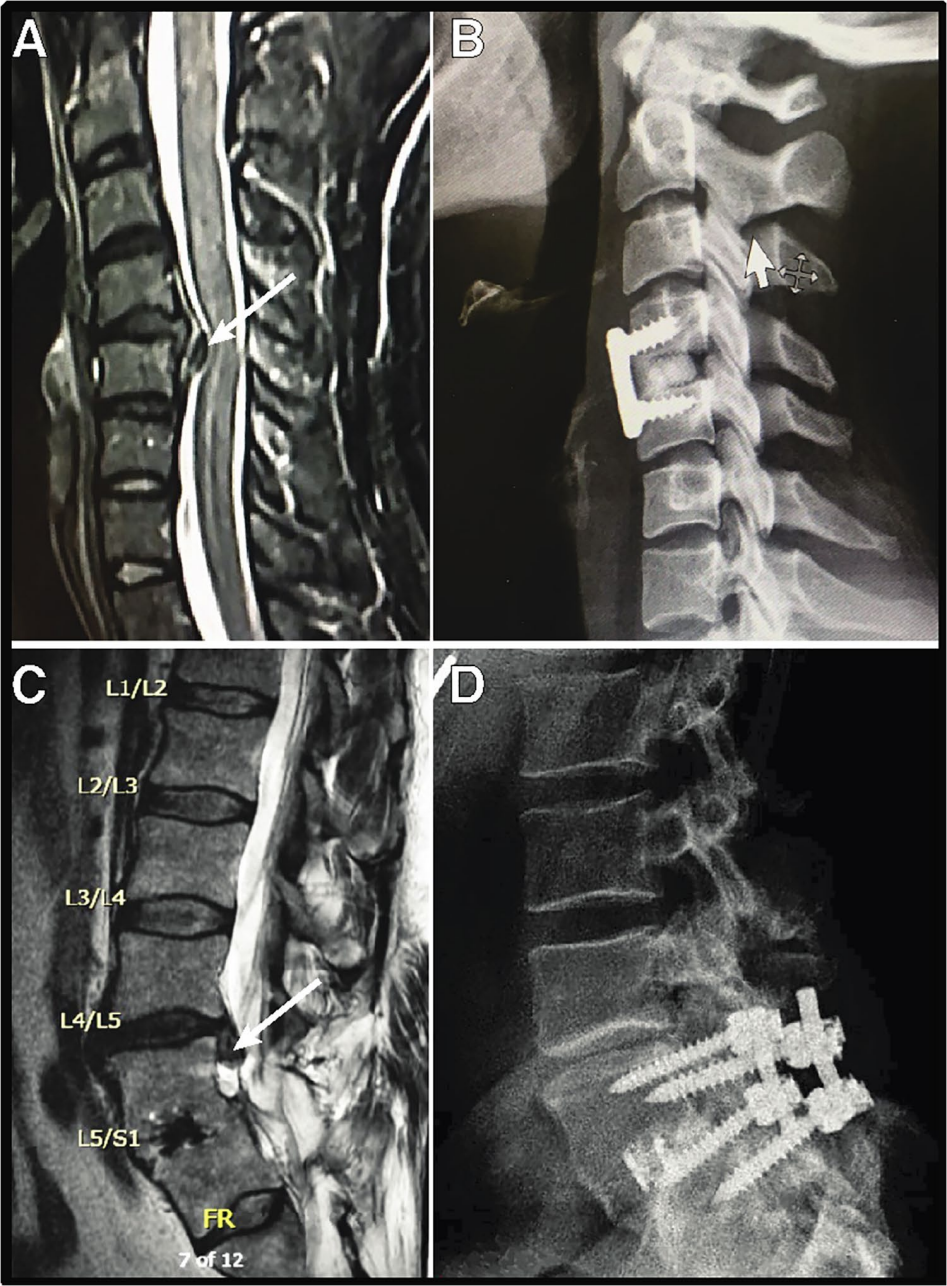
A MRI of the cervical spine of CRM device representative only a few years after starting employment. Arrow points to the cervical spine disc herniation. B Lateral spine x-ray following repair of herniation in A. C MRI of the lumbar spine of electrophysiologist with new disc herniation at L4-L5. A prior repair is seen with fusion and titanium cage at L5-S1 that was required approximately 5 years after starting practice. D Sagittal view of the lumbar spine of C
